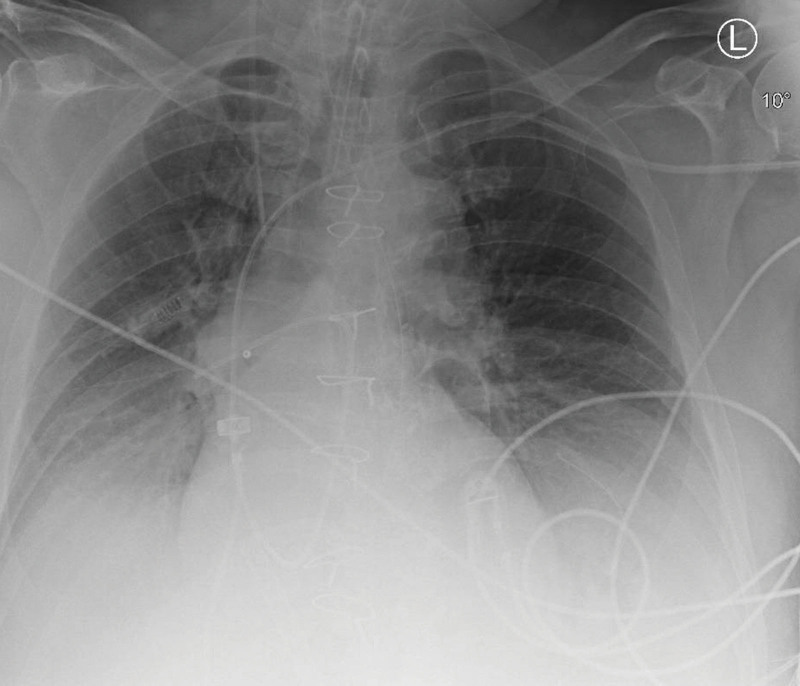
A chest x-ray taken on the day of the measurements, at 10 cm H_2_O of PEEP. PEEP, positive end-expiratory pressure.
